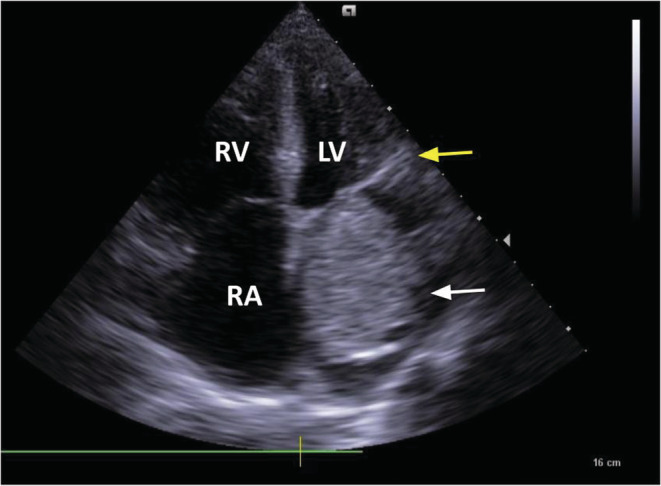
Transthoracic echocardiogram showing a giant mass (white arrow) in the left atrium obstructing the mitral valve (yellow arrow) that is suggestive of myxoma
